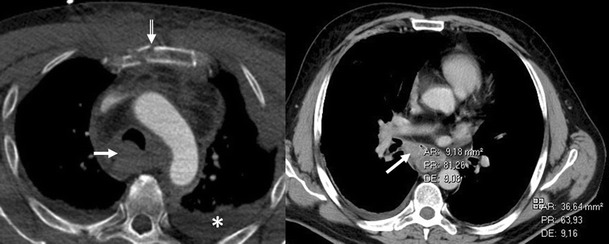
a Contrast-enhanced CT scan of a man who had suffered a traffic accident. An infiltrative mediastinal haematoma is identified with subtle areas of high CT-attenuation values (*arrow*). Bilateral pleural effusion (*) and a sternum fracture (*open arrow*) are also observed. b Iatrogenic mediastinal haematoma (*arrow*) in a 64-year-old man secondary to bronchoscopy with transtracheal biopsy. Note the high attenuation value of the lesion compared with muscular tissue
